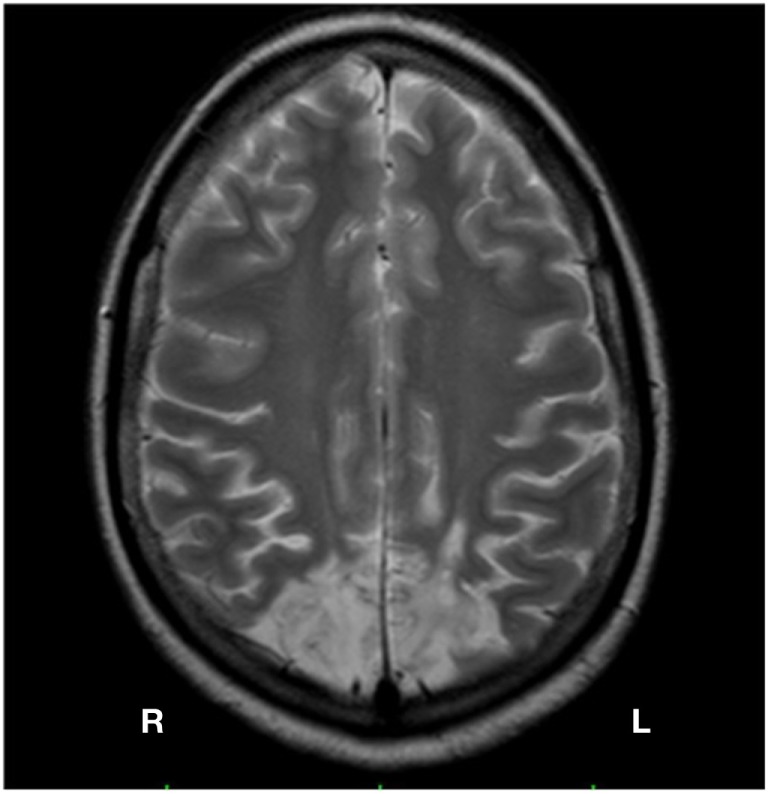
Brain MRI of GP Bilateral lesion of the occipital cortex, slightly greater on the right side, with involvement of the adjacent parietal lobes.
